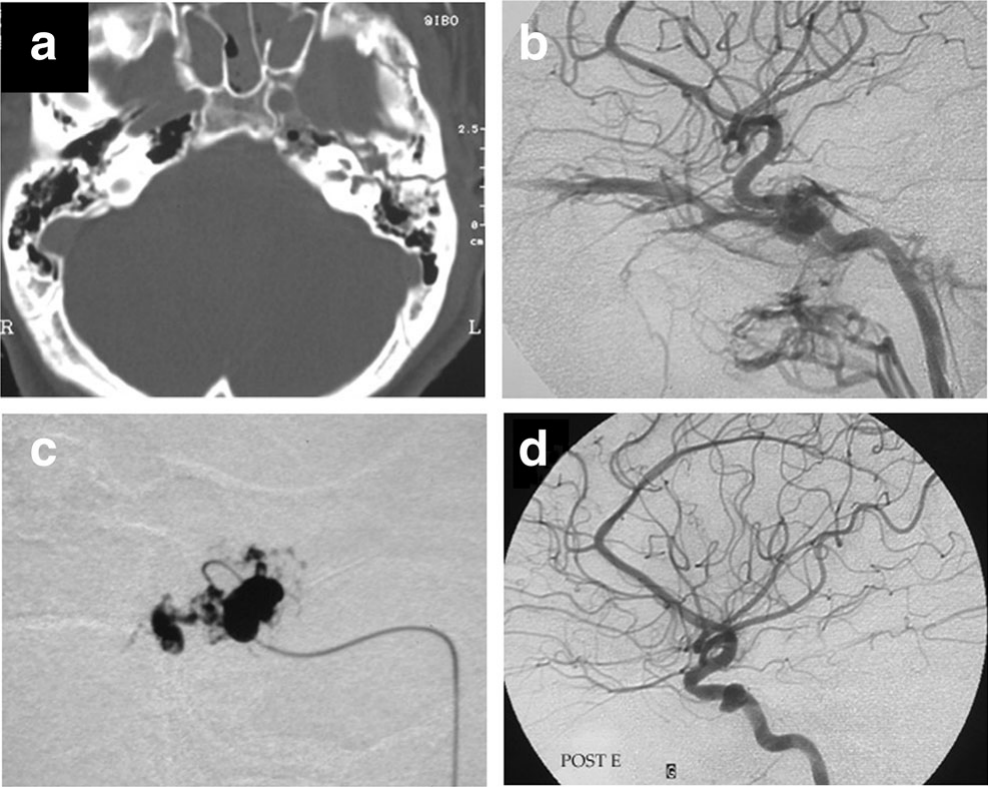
16-year old male patient suffering a complex compound skull base fracture after a motor vehicle accident (CT-scan, a), presenting with 1-month history of pulsatile exophthalmus. b Initial DSA demonstrates high-flow CCF with prominent venous hypertension. c With a precise NBCA injection in the cavernous sinus at the fistulous point, the CCF is closed. d Late follow-up control angiogram demonstrating full occlusion of the CCF, patent ICA, and the classical pouch often seen at the fistulous communication after cure. The small residual pouch seen in panel d did not warrant further treatment.
